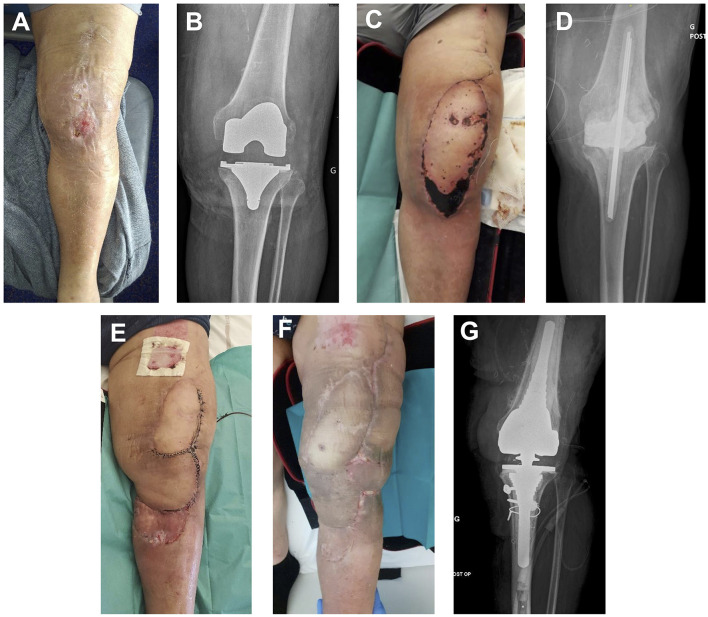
(A) Initial presentation after 10 days of ceftobiprole for left knee cellulitis. (B) X-ray at initial presentation. (C) Thigh flap partial necrosis 10 days after implant removal surgery. (D) X-ray after implant removal surgery. (E) Second surgery: spacer replacement, rotation flap, and STSG. (F) Clinical presentation 2 months after last surgery (total knee arthroplasty reimplanted). (G) X-ray at 12 months follow-up after total knee arthroplasty reimplantation.
